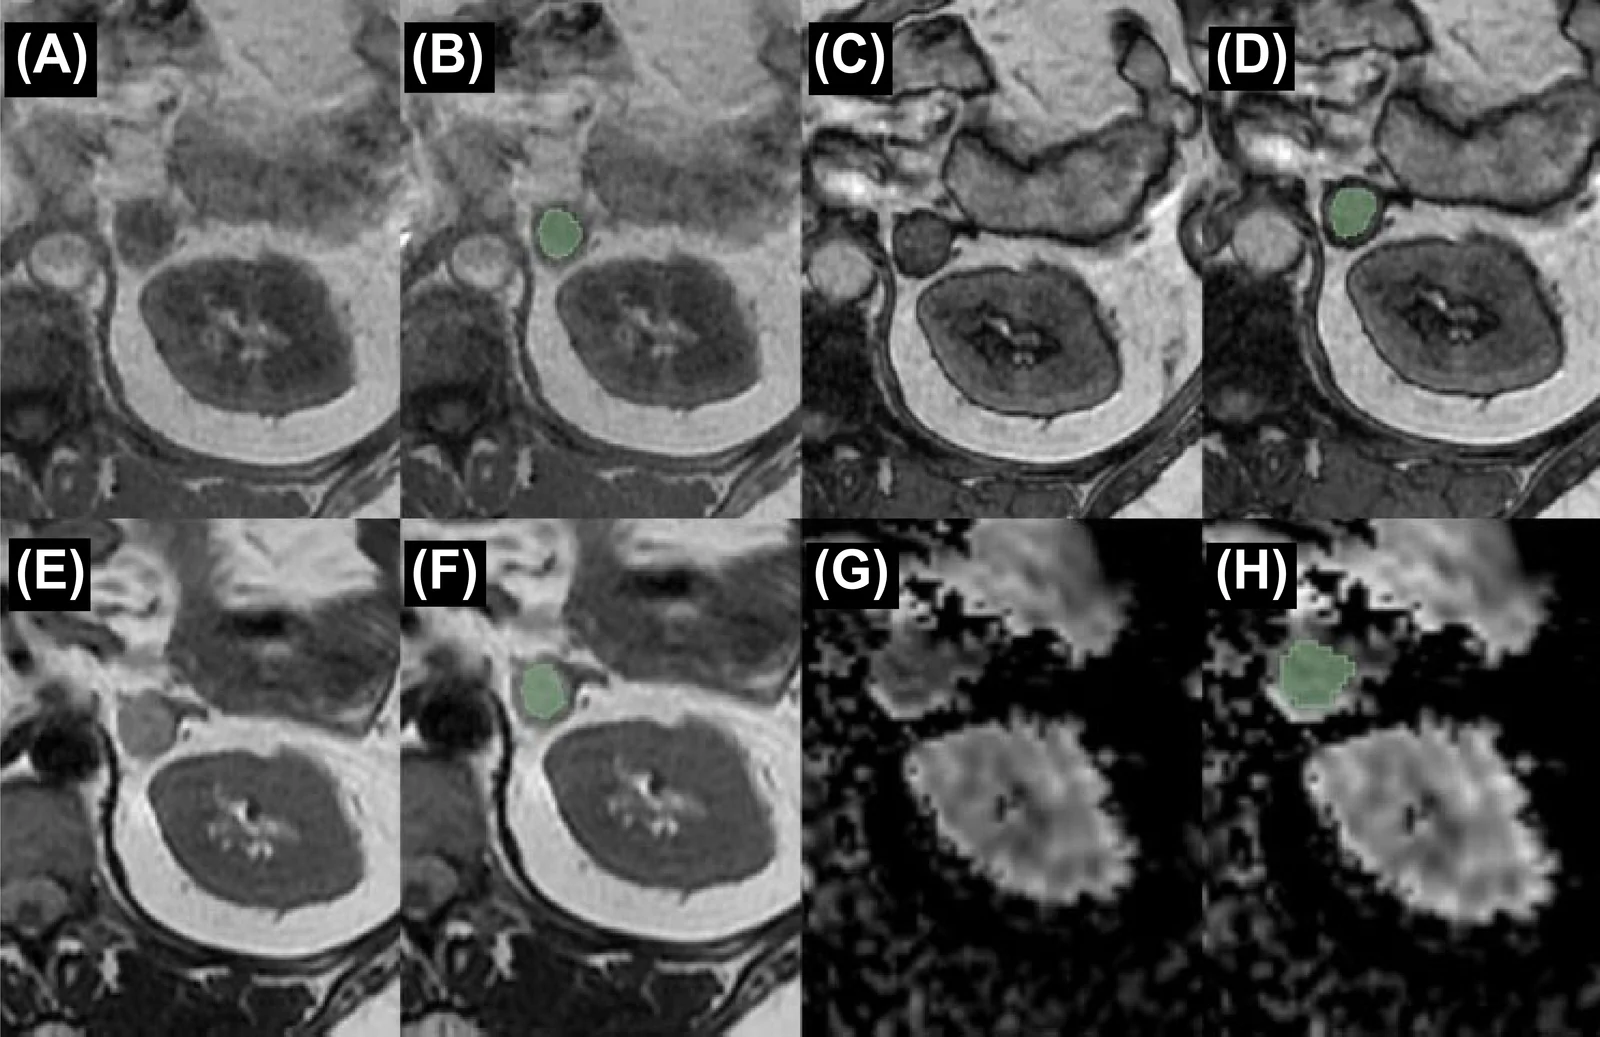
Segmentation process. In-phase chemical shift imaging (CSI) (A, B), opposed-phase CSI (C, D), T2-weighted image (E, F), and apparent diffusion coefficient map (G, H).
